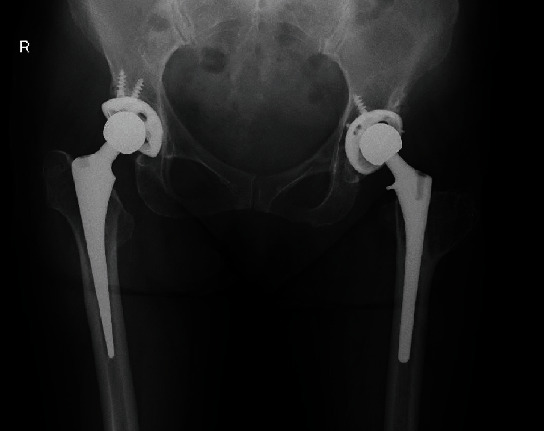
Radiographs of Case 2 at their last follow-up (11 years) after total hip arthroplasty.
